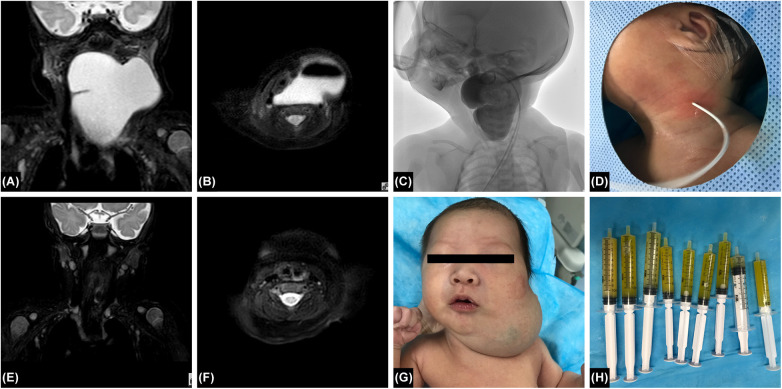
(A) Pre-treatment coronal T2-weighted MRI image showing a large, well-defined hyperintense lesion in the head and neck region, suggestive of lymphatic malformation with cystic fluid accumulation. (B): Pre-treatment T2-weighted MRI image showing a large lesion compressing surrounding tissues. (C): Intracavitary view after percutaneous puncture during treatment, with pigtail drainage catheter in place. DSA single-frame fluoroscopic image demonstrating contrast agent diffusion within the lesion cavity, clearly delineating its morphology and extent. (D): Intraoperative view showing the puncture needle placement site for percutaneous insertion into the lesion cavity. (E): Follow-up coronal T2-weighted MRI after pingyangmycin and 1% lauromacrogol alternating sclerotherapy shows significant reduction in lesion volume, near-complete disappearance of the hyperintense cystic cavity, and normalization of residual tissue signal. (F): Post-sclerotherapy T2-weighted MRI demonstrates near-complete resolution of the lesion with clear visualization of surrounding tissues. (G): Pre-treatment clinical photograph showing diffuse swelling of the head and neck, with facial deformity due to lesion compression. (H): Pale yellow lymphatic fluid aspirated.
